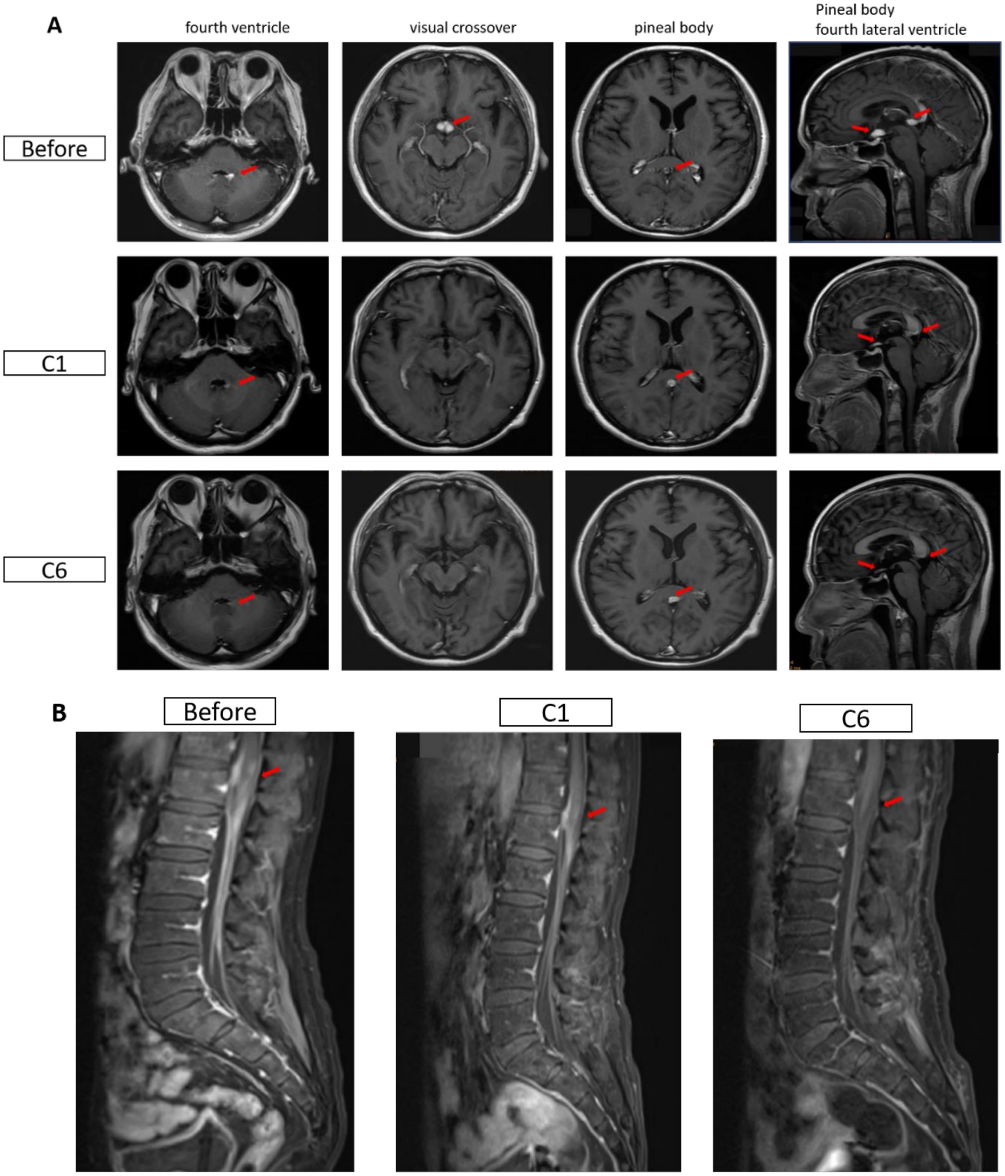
Magnetic resonance imaging (MRI) images of the head in both patients. (A) Changes in cranial MRI before and after treatment for Case 1. Before: April 7, 2024; C1: May 6, 2024; C6: August 15, 2024. (B) Changes in the conus and cauda equina regions before and after treatment for Case 2. Before: May 25, 2024; C1: June 26, 2024; C6: November 14, 2024. The red arrows indicate the lesions.
